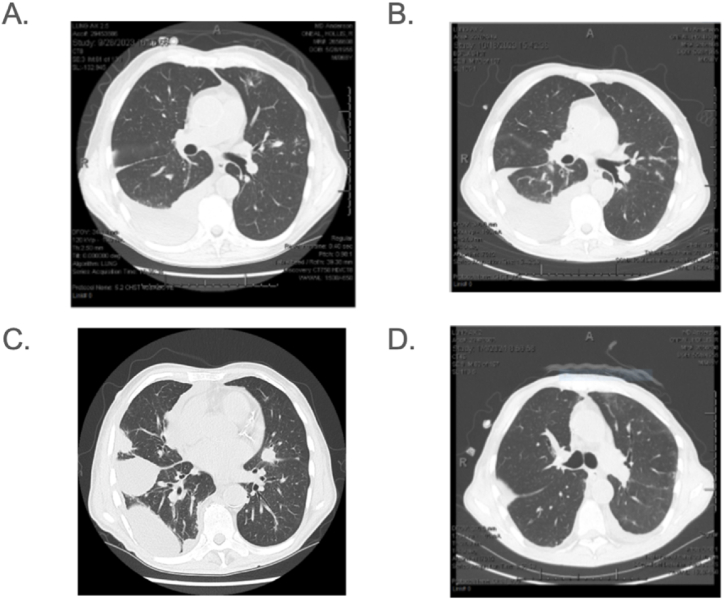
A. CT chest without contrast from 37 days post-transplant and after initial treatment for fungal pneumonia was initiated; B. CT chest without contrast from 65 days post-transplant; C. CT chest without contrast from 80 days post-transplant prior to placement of chest tubes for empyema; D. CT chest without contrast from 143 days post-transplant showing resolution of right sided pleural effusion.
